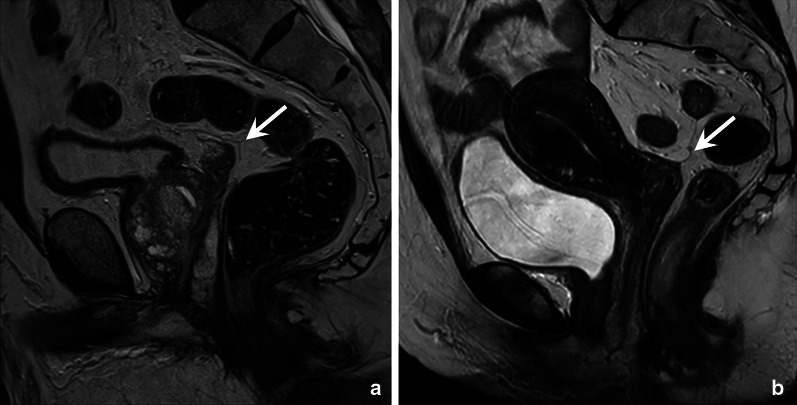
Sagittal T2-weighted MR images demonstrating the anterior peritoneal reflection in a male individual (without rectal cancer), at the top of the seminal vesicles (a) and in a female (without rectal cancer) where it is located at the level of Douglas’ pouch (b). The peritoneal reflection can be recognised as a thin V-shaped “fold” (arrows)
